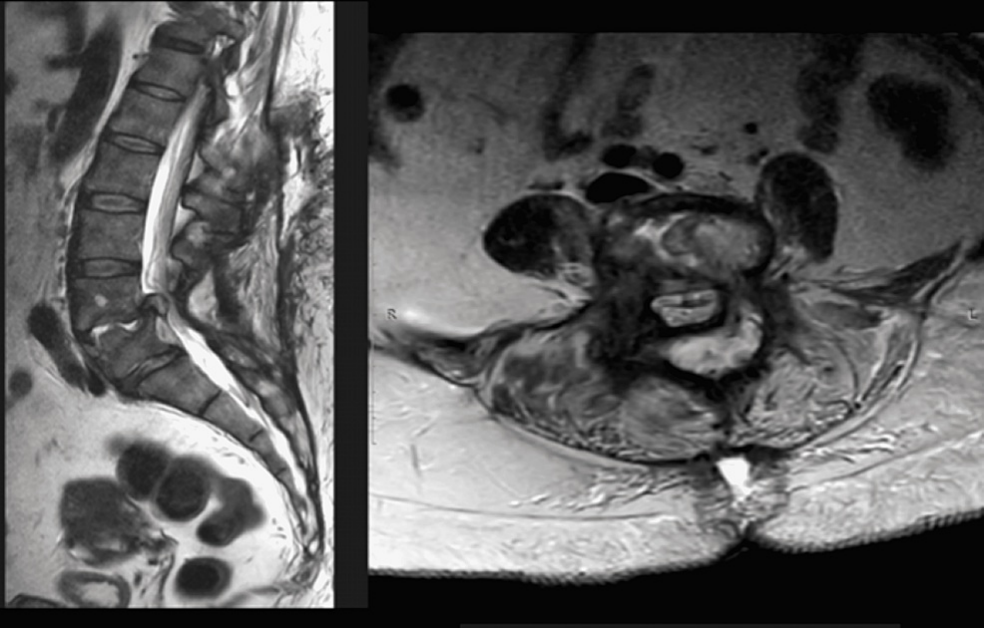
Preoperative of the first surgical stage, from left to right: sagital and axial MRI slides on T2. It is observed a remarkable stenosis narrowing and compressing the spinal canal, and stenosis and the infectious process extending from the ventral part of the vertebral body at the level L4-L5.
